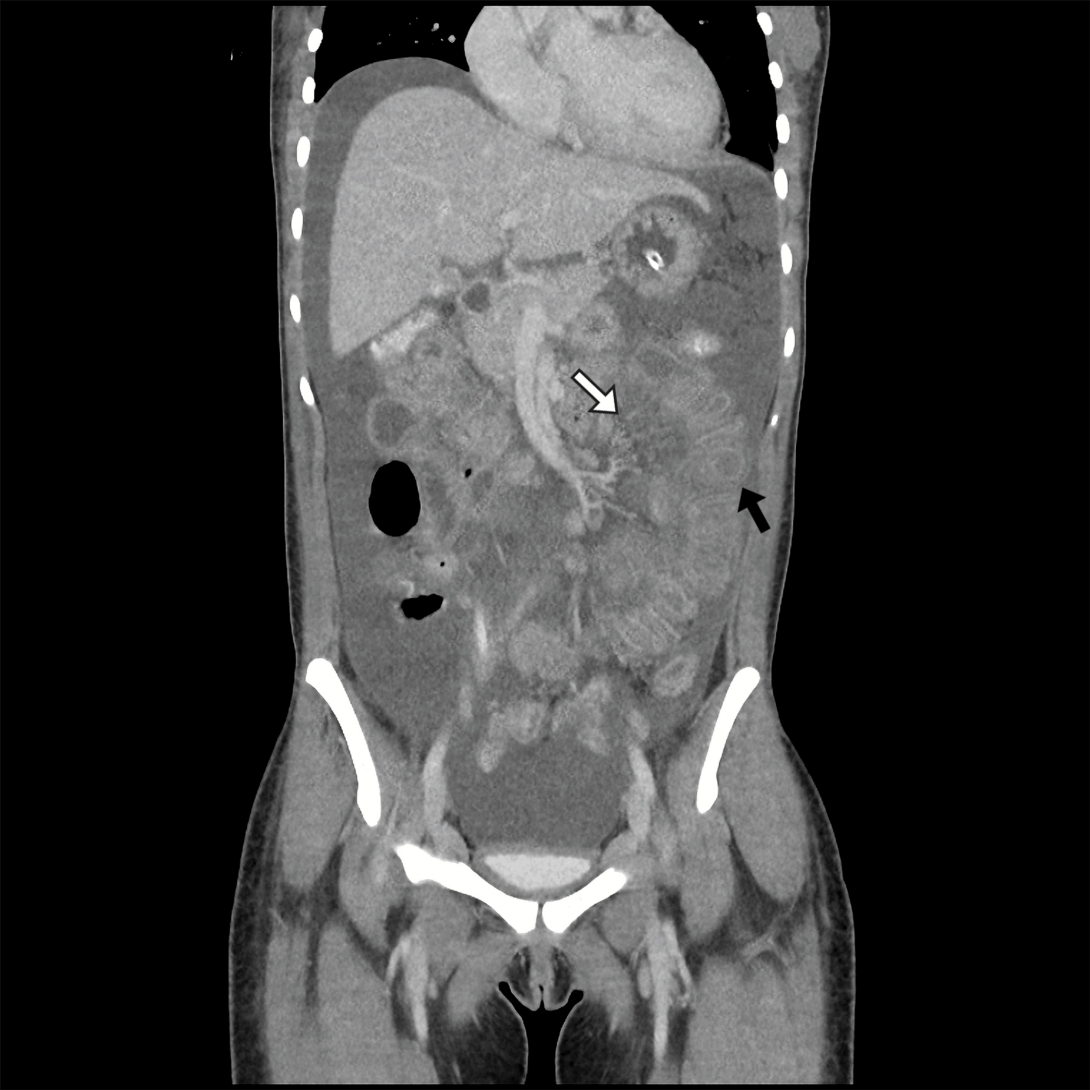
Coronal venous-phase contrast-enhanced abdominopelvic computed tomography (CT) image. This study demonstrates diffuse, circumferential thickening of multiple small-bowel loops, predominantly involving the jejunum and ileum, associated with marked submucosal edema, resulting in a characteristic “target” or “double-halo” appearance of the transversally seen bowel loop (black arrow). These findings are accompanied by mesenteric fat stranding and vessel engorgement, also referred to as a "comb sign" (white arrow). A moderate amount of free intraperitoneal fluid is also noted. As a whole, these findings can be attributable to an inflammatory small bowel process, in this case an active lupus enteritis.
